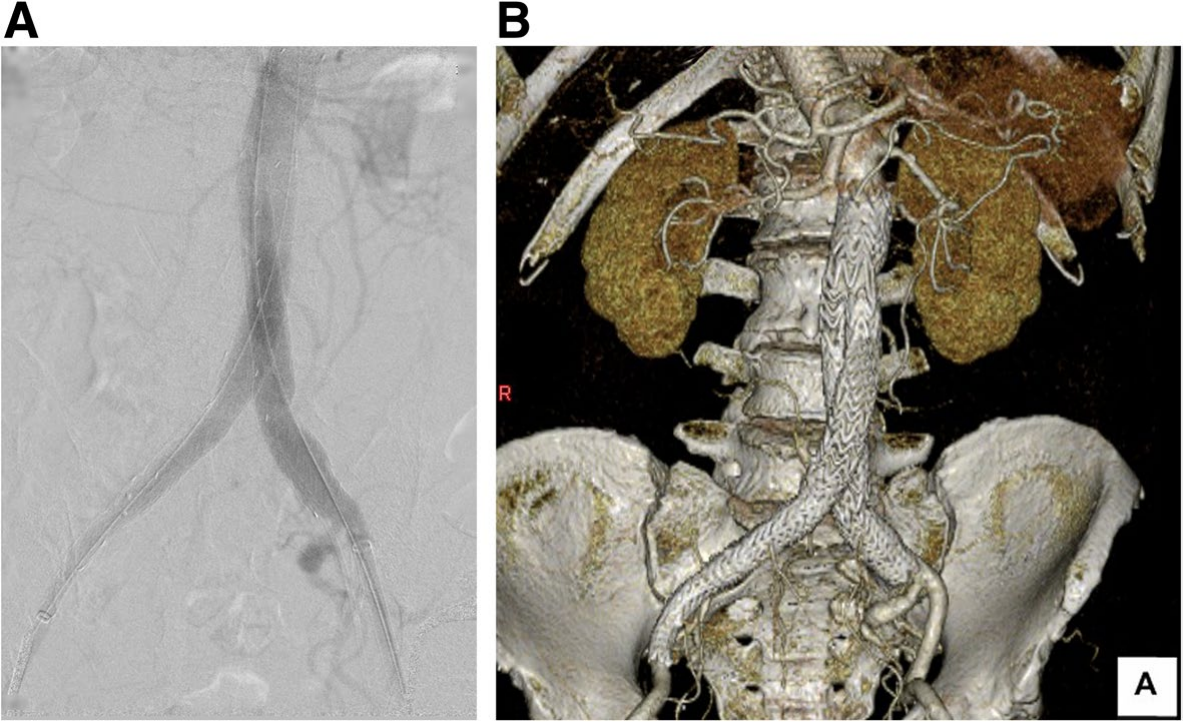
Final intraoperative angiography and postoperative three-dimensional reconstruction of computed tomography angiography findings A Intraoperative final angiography confirming the absence of persistent arterio-venous fistula and significant endoleak. B Postoperative three-dimensional reconstruction of computed tomography angiography performed one month after surgery showing complete aneurysm exclusion and fistula dissipation
